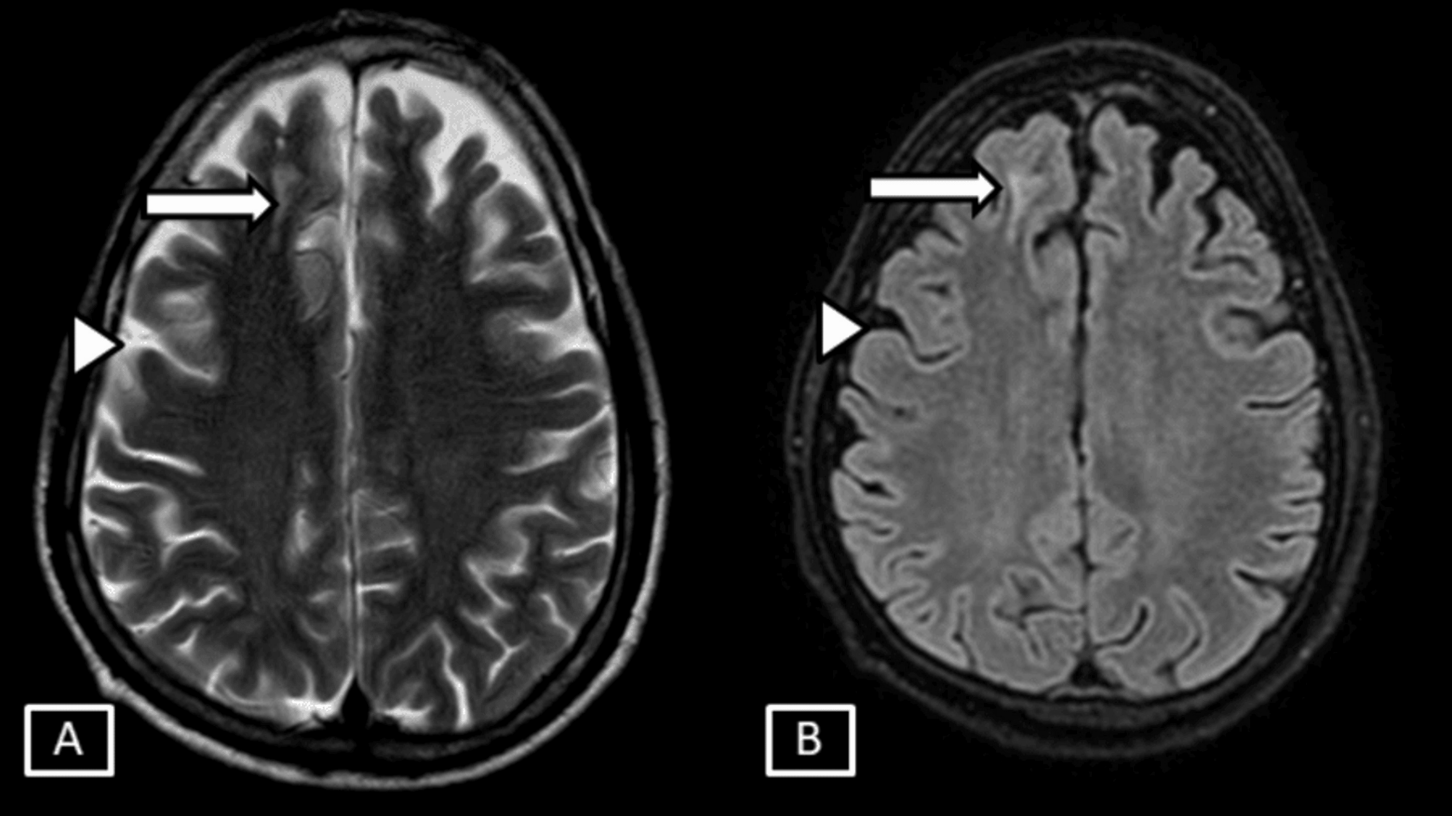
Case 1 MRI T2 axial (A) and T2/FLAIR axial (B). Images show thin linear T2/FLAIR hyperintense signal changes involving the juxtacortical areas of the right frontal lobe represented by the white arrows. Age related brain parenchymal atrophic changes in the form of prominent extra axial sulcal spaces represented by white arrowheads. FLAIR: Fluid Attenuation Inversion Recovery
